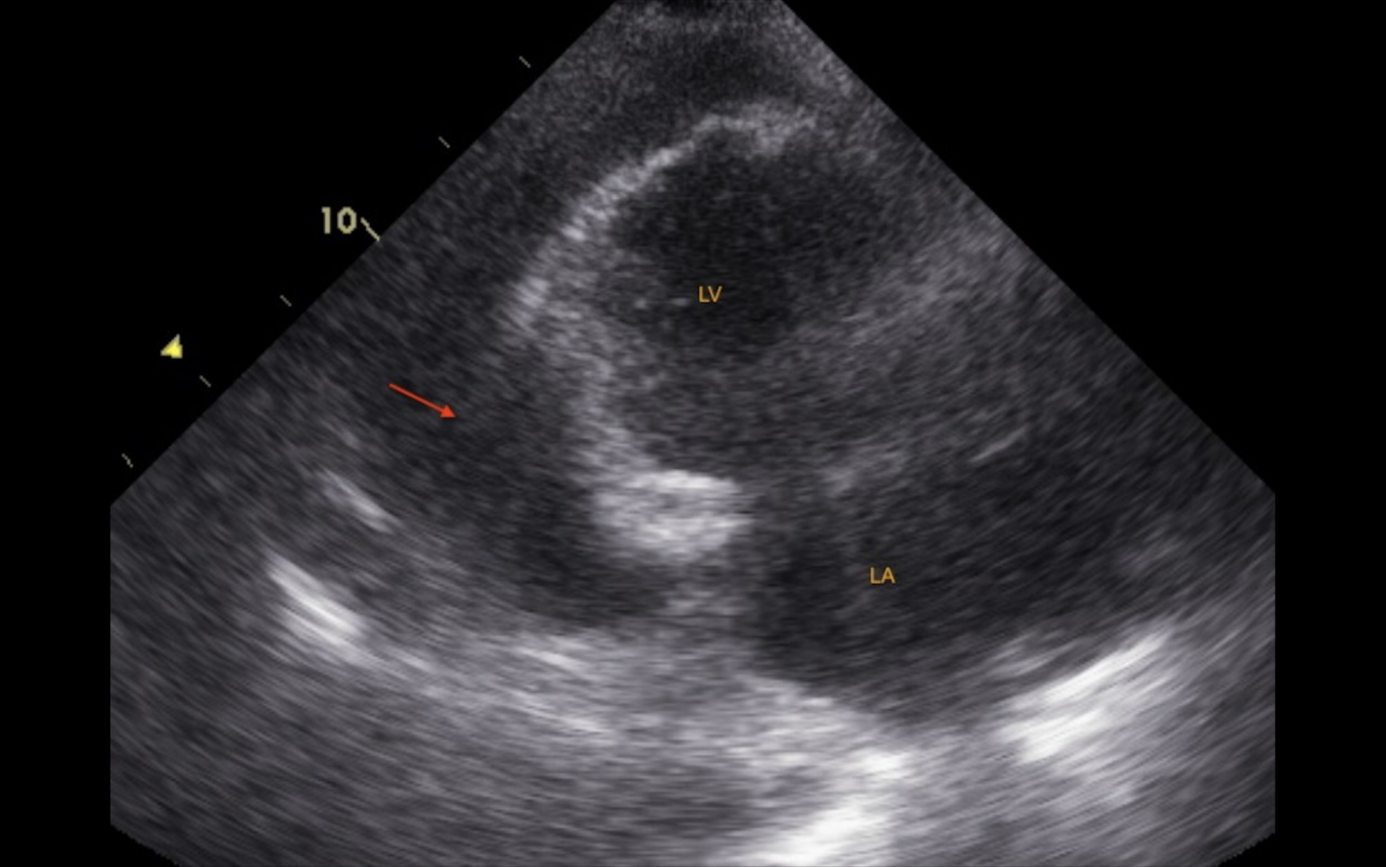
Transthoracic echocardiogram: apical two-chamber view The image shows large circumferential pericardial effusion, predominantly posterior in a location with fibrinous strands (arrow) LA: left atrium; LV: left ventricle
